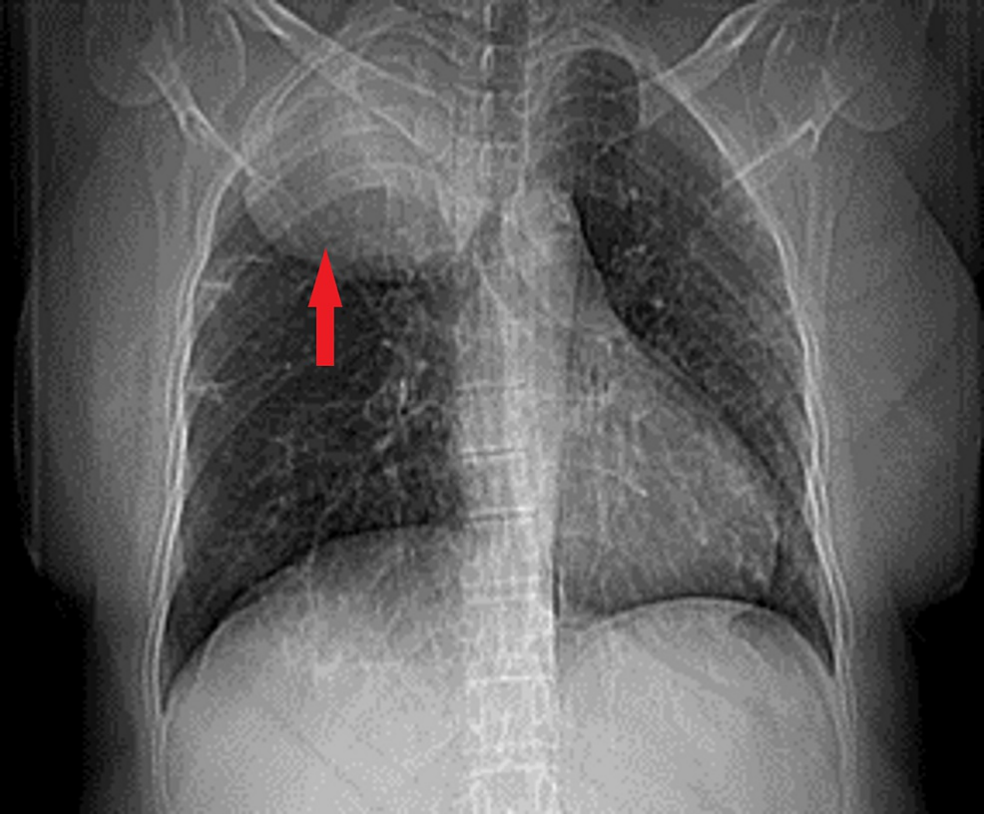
Chest x-ray on presentation showing a large right upper lung lobe mass, slightly impinging on the trachea (marked with red arrow).
